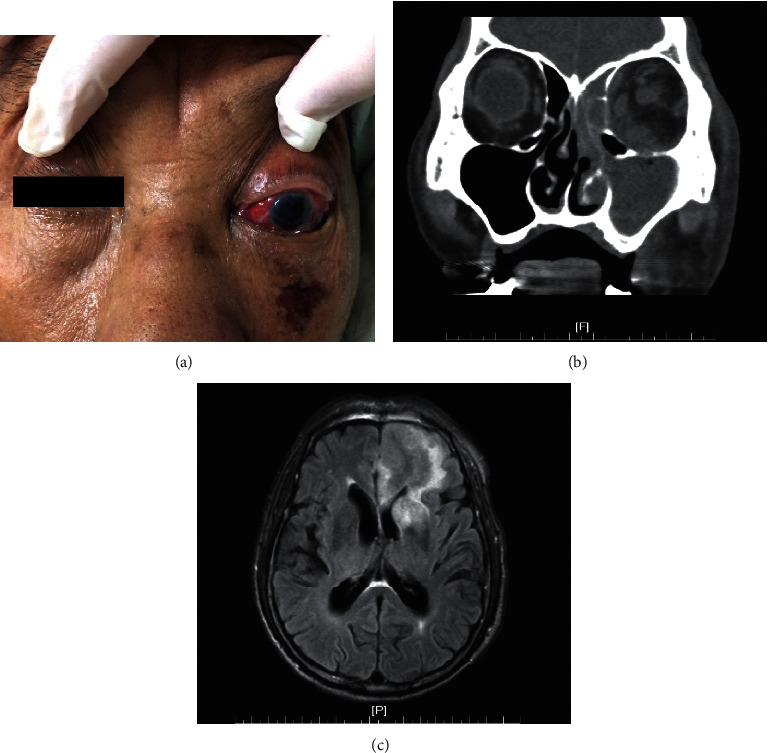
This was a 73-year-old male patient. He died 22 days after disease onset. The culture reported *Rhizopus*. (a) The patient presented as left facial swelling and tenderness, left eye proptosis, painful extraocular movement, eyelid swelling and erythema, conjunctival injection and chemosis, and cornea edema. (b) Computed tomography revealed orbital intraconal infiltration, extraocular muscles swelling, and sinusitis over maxillary and ethmoid sinuses. (c) Magnetic resonance imaging was performed 2 days after the CT, which revealed ipsilateral brain edema with midline shift.
